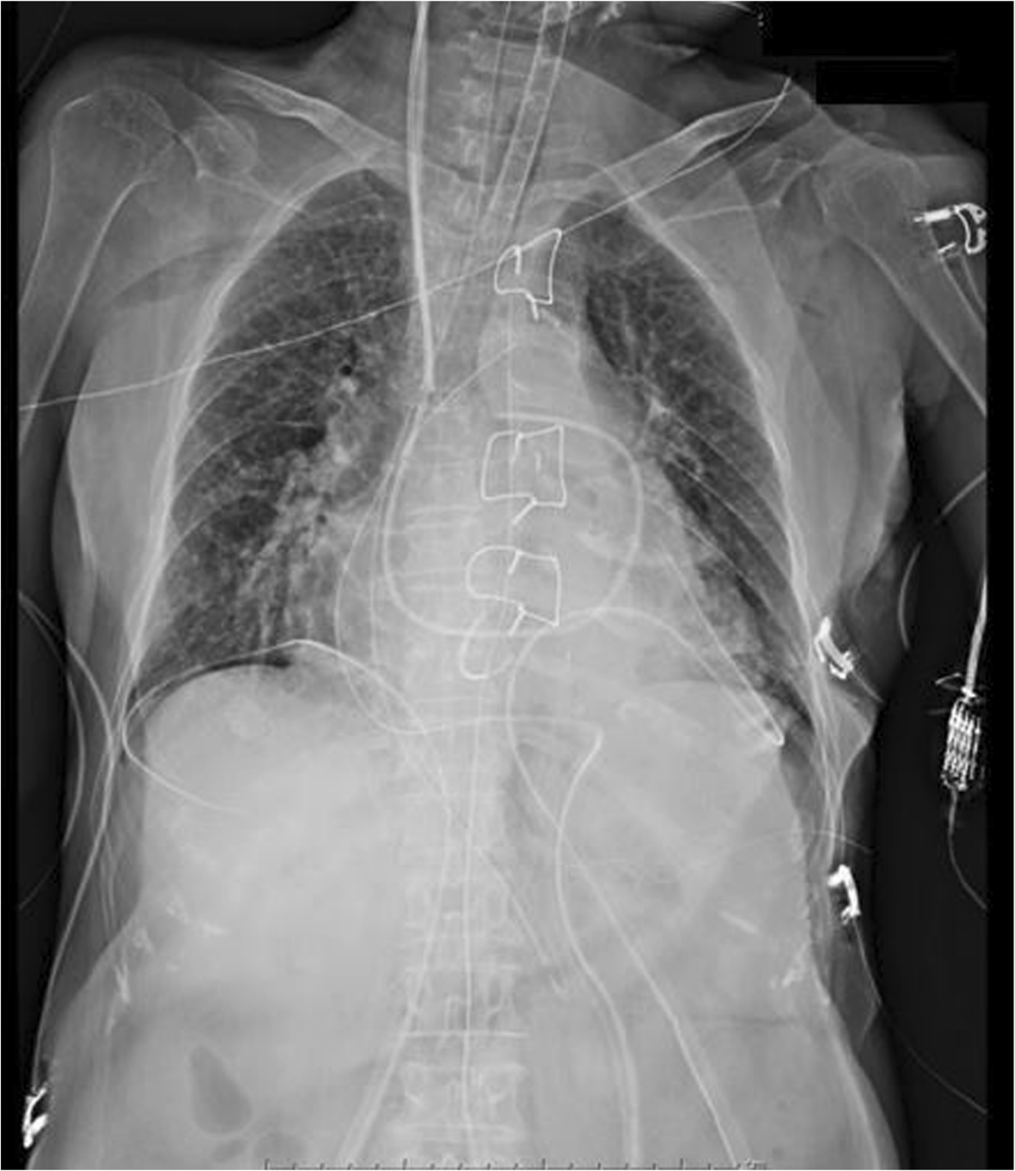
Chest radiography just after the cardiac surgery. The course and the tip position of the NG tube were unusually shifted to the right
